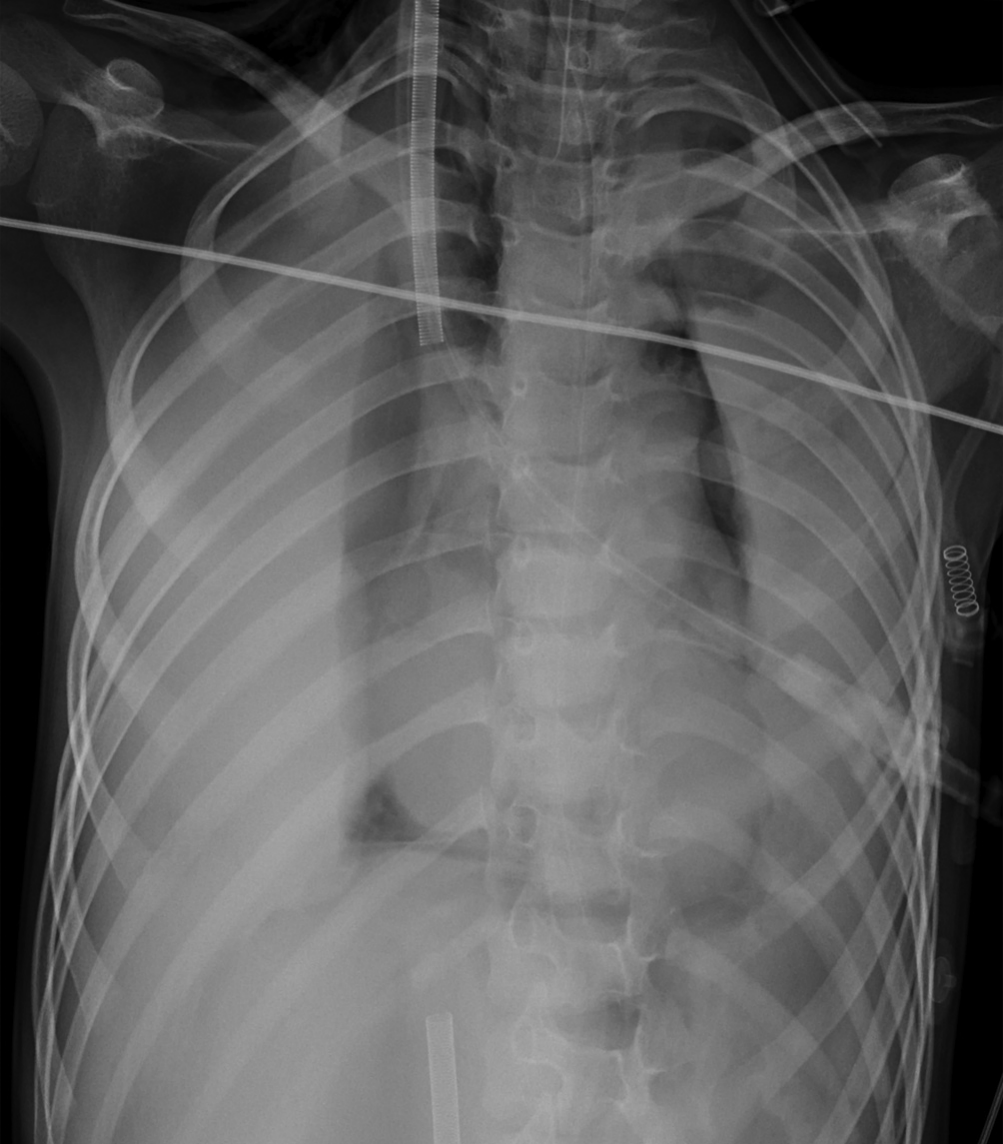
Case x-ray performed 42 h after ECMO initiation.
